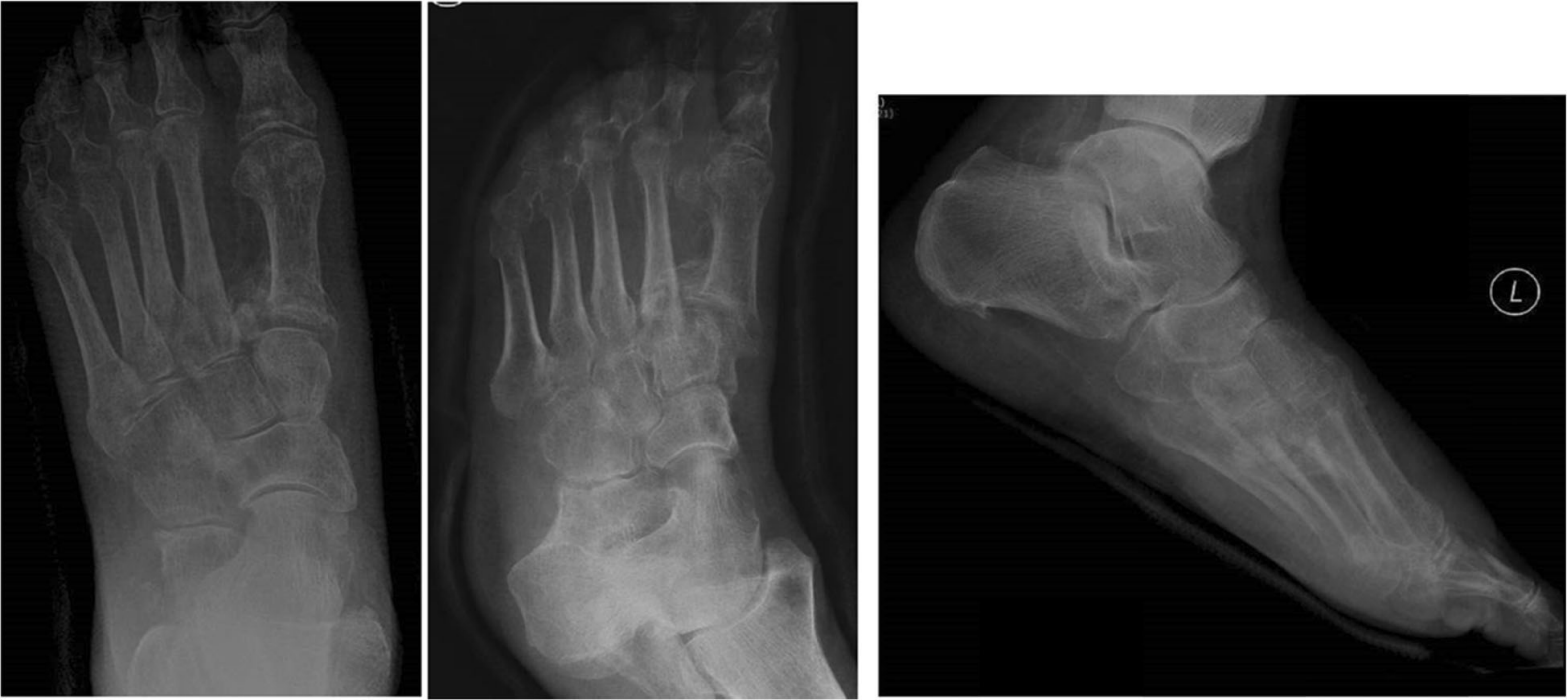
Radiograph of participant who scored a mean of 7.2 out of 11 on the severity scale and rated their quality of life 95/100
